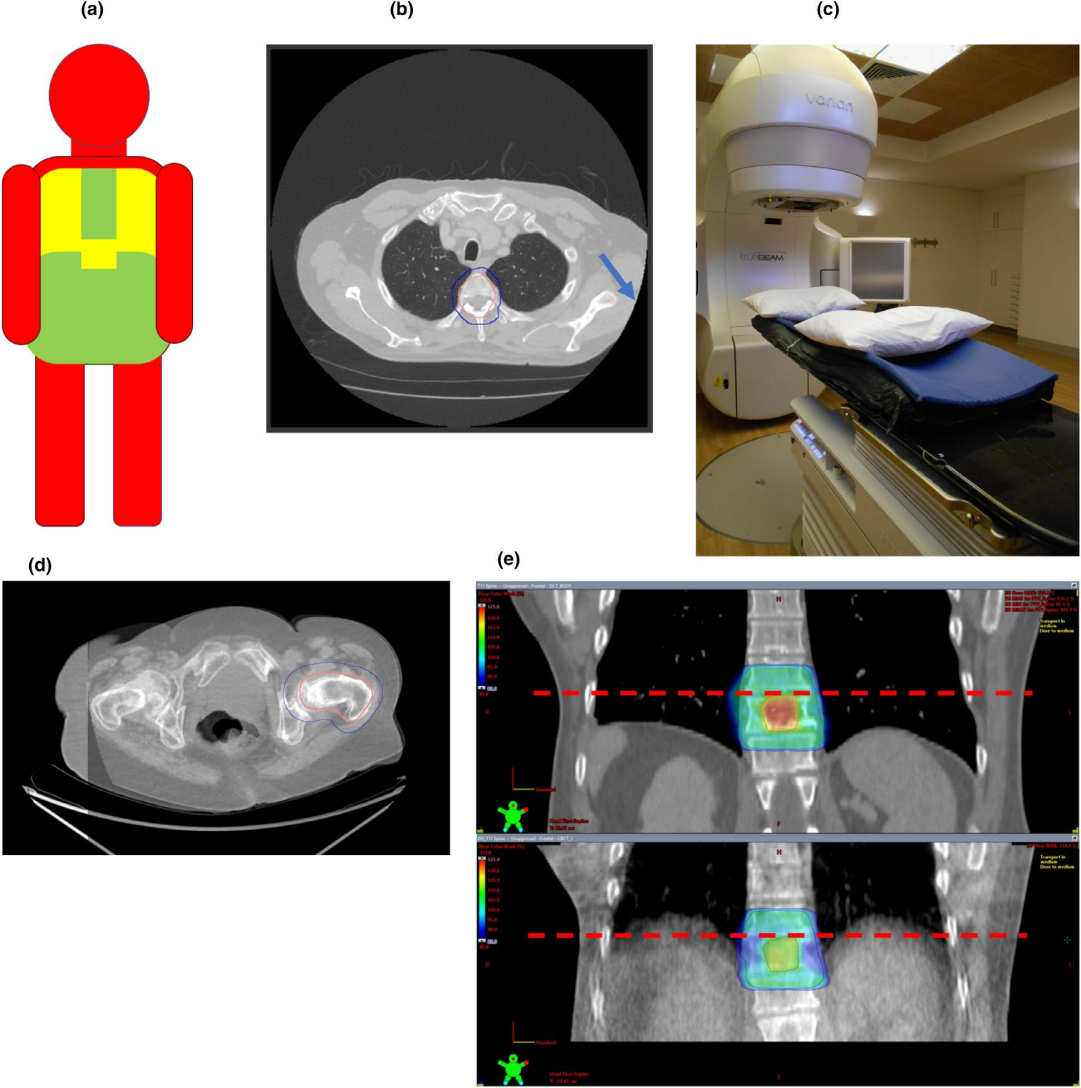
(a) Representation of the suitability of different treatment sites for SFRT. Areas shaded in green usually reproduce well from the dCT to treatment; areas in yellow are more variable, and so more thought is required, and areas in red are generally considered particularly variable and therefore unsuitable. (b) Adequate FOV for adequate visualisation and calculation. Note that some image deficit (indicated with a blue arrow) may be acceptable for SFRT planning, depending on the clinical situation—e.g., treatment angles could be chosen to avoid this area. (c) SFRT is set up with a curved full‐body vacuum bag. Using curved vacuum bags during treatment aims to more closely replicate the patient's planned dCT positioning on the treatment couch. (d) An example of a treatment verification CBCT: Utilising daily CBCT scans to verify and correct positioning gives confidence in the SFRT treatment delivery. Note the good agreement within and around the target regions in this example, despite discrepancies in less relevant areas. (e) An example of variation in diaphragm position on a T Spine plan. The dCT image (top) is in breath hold (note the clear delineation of the diaphragm in its stable position). The CBCT image (bottom) is free‐breathing (note the average position of the diaphragm) and results in a cooler distribution in this area.
